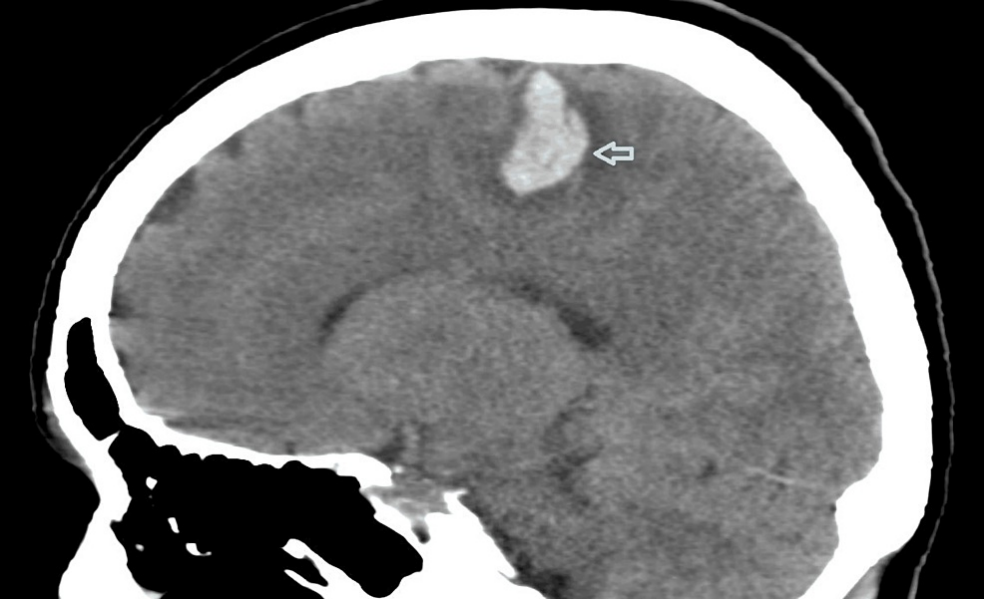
CT Head saggital view at admission shows parenchymal haematoma in right precentral gyrus and posterior aspect of right superior frontal gyrus
